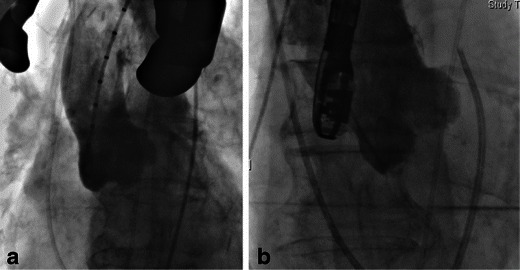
Intraprocedural fluoroscopy. Procedural fluoroscopy demonstrating a pigtail catheter in the non-coronary cusp of the aortic root, with aortography demonstrating the sinus of Valsalva and ascending aorta. The pigtail catheter is used for intermittent aortography during the procedure to aid in correct device positioning and also to determineTransoesophageal haemodynamics and degree of aortic incompetence. a Baseline aortography during a direct aortic access procedure (incision ‘spreaders’ shown). Aortography aids in identification of the optimal position for aortic puncture needed to introduce the large-bore arterial access sheath. b Baseline aortography with Swan-Ganz catheter in situ in the pulmonary artery from right internal jugular access and transesophageal echocardiography probe
